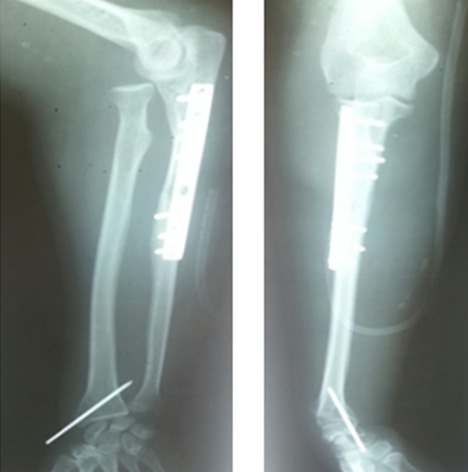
Postoperative radiographs showing a good reduction of the fracture but persistent anterior dislocation of the radial head
